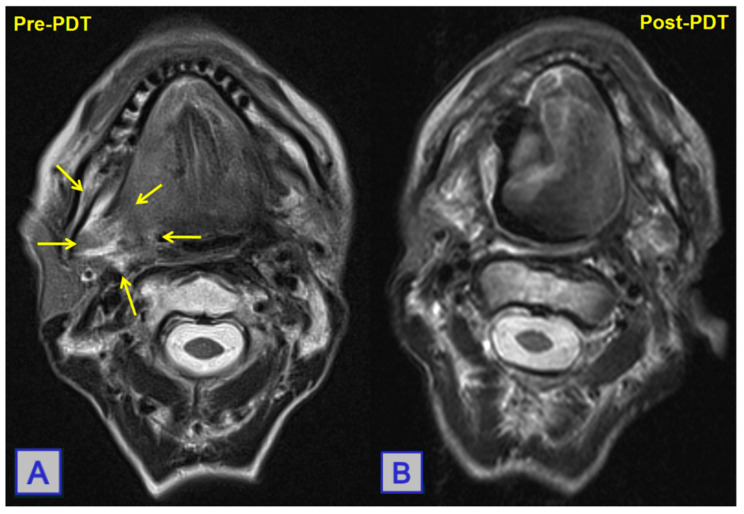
MRI showing a posterolateral tongue-base squamous cell carcinoma. Pre-PDT (A), a large mass is seen. Post-PDT (B), a reduction in lesion size with widespread tumour necrosis is noted. Reprinted with permission from ref. [10]. Copyright 2010 Clin. Oncol. (R. Coll. Radiol.).
